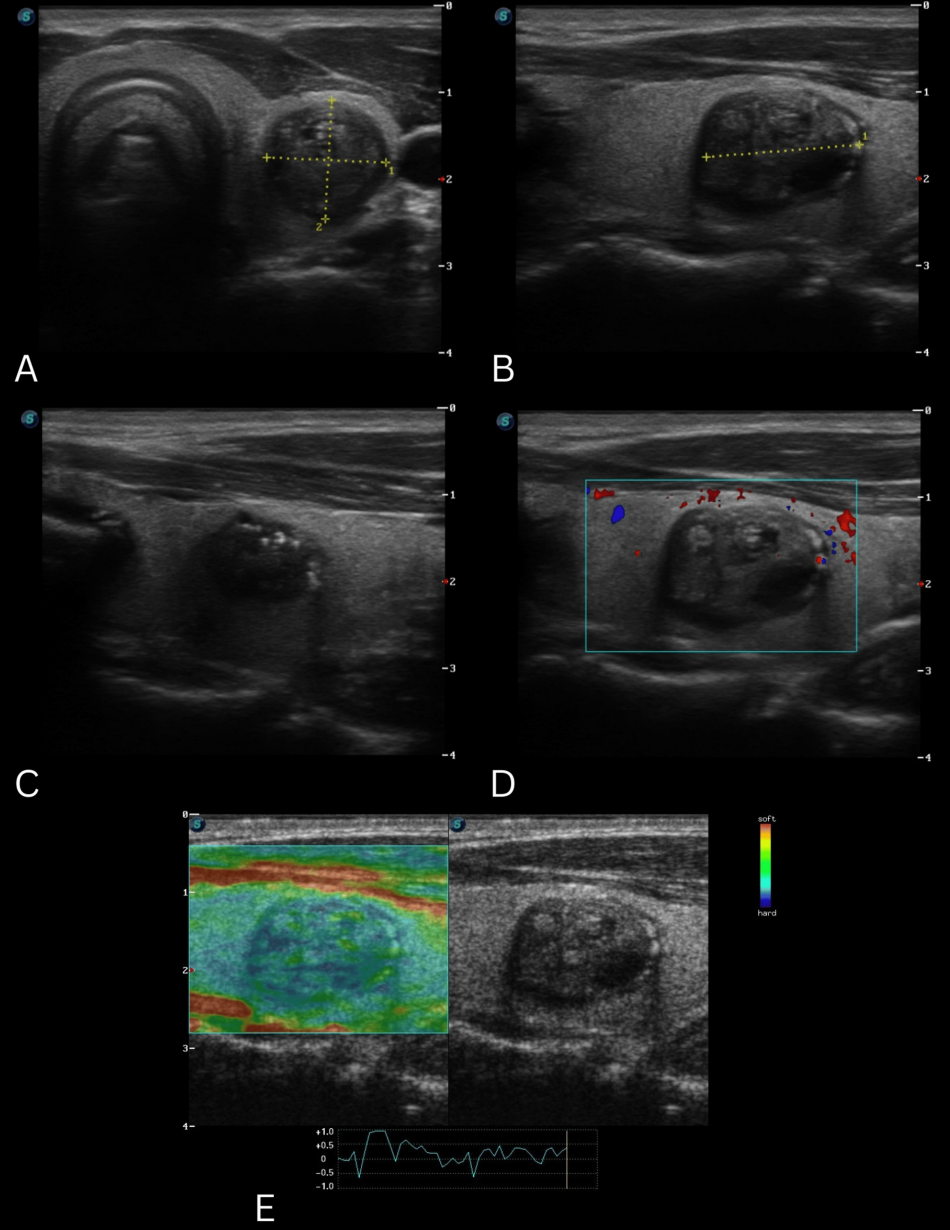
Clinical images series of TUSG 2 A. Nodular lesion in the left thyroid lobe, solid (2), hypoechoic (2), regular margins (0); B. Wider-than-tall (0); C. Incomplete eggshell calcification with irregular acoustic shadowing at the margins (2); D. Absence of peripheral vascularity on color Doppler, short axis; E. Increased stiffness on elastography under compression; TIRADS-4 (6 points) 13.76 x 13.19 x 17.72 mm TSUG: thyroid ultrasonography; TIRADS: Thyroid Imaging Reporting and Data System
